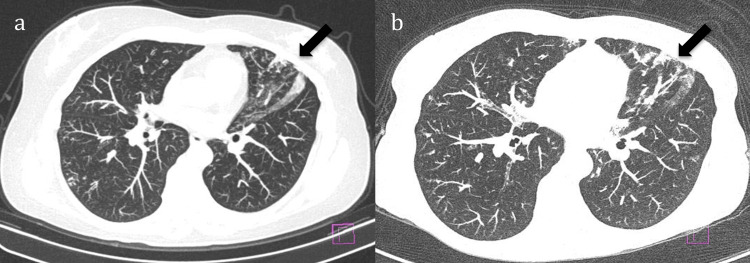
Lung interstitial findings (arrows) from chest CT scans obtained in 2016 (a) and 2024 (b)
